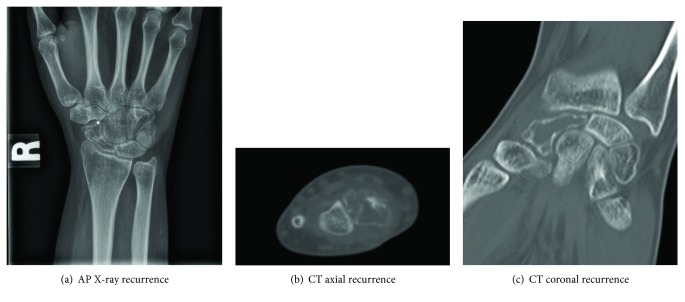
(a–c) AP radiograph (a) and axial and coronal CT scans (b, c) of the right wrist, demonstrating increased lucency and graft resorption within the scaphoid, consistent with local recurrence of the giant-cell tumor of bone.
